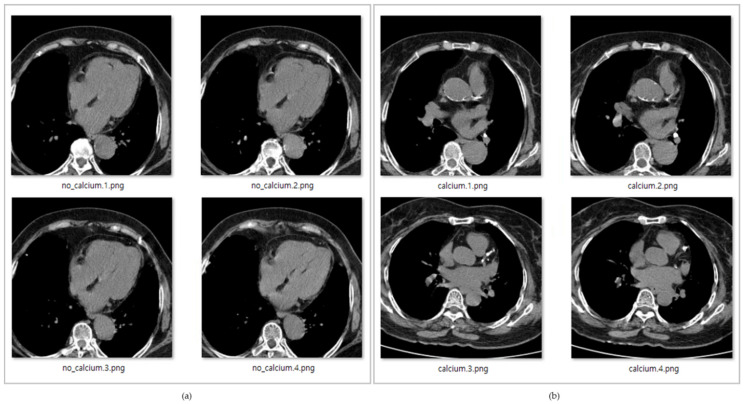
(a) Original coronary artery calcium score CT image without calcification, (b) Original coronary artery calcium score CT image with calcification.
